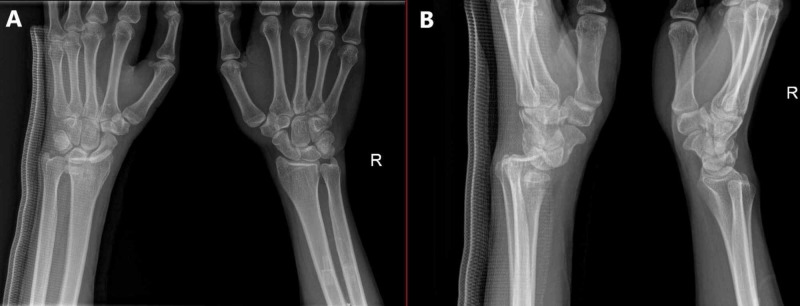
X-ray of the left wrist (injured) and contralateral wrist. A) Anteroposterior (AP) view ; B) Lateral view
